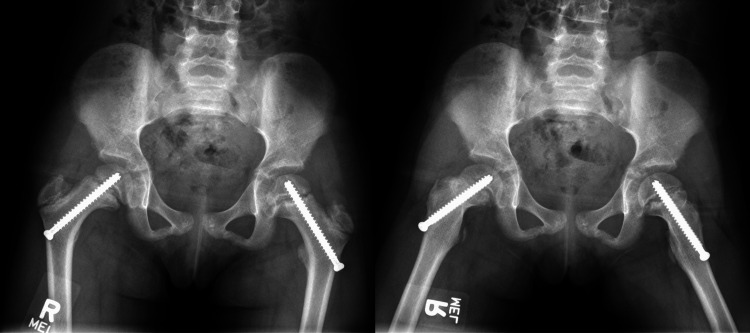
Anterioposterior and frog leg X-rays at the four-month follow-up demonstrating mild epiphyseal migration and growth along cannulated screws.
